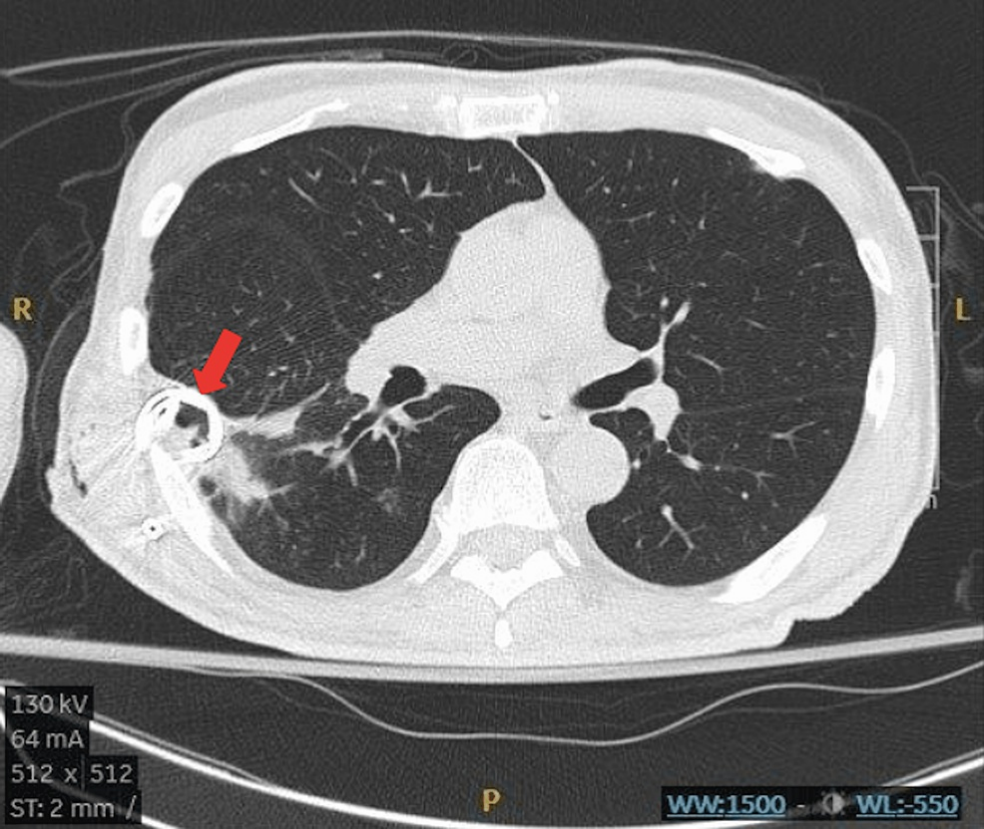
CT scan of the abscess post-catheter insertion. The red arrow points to the draining catheter.
